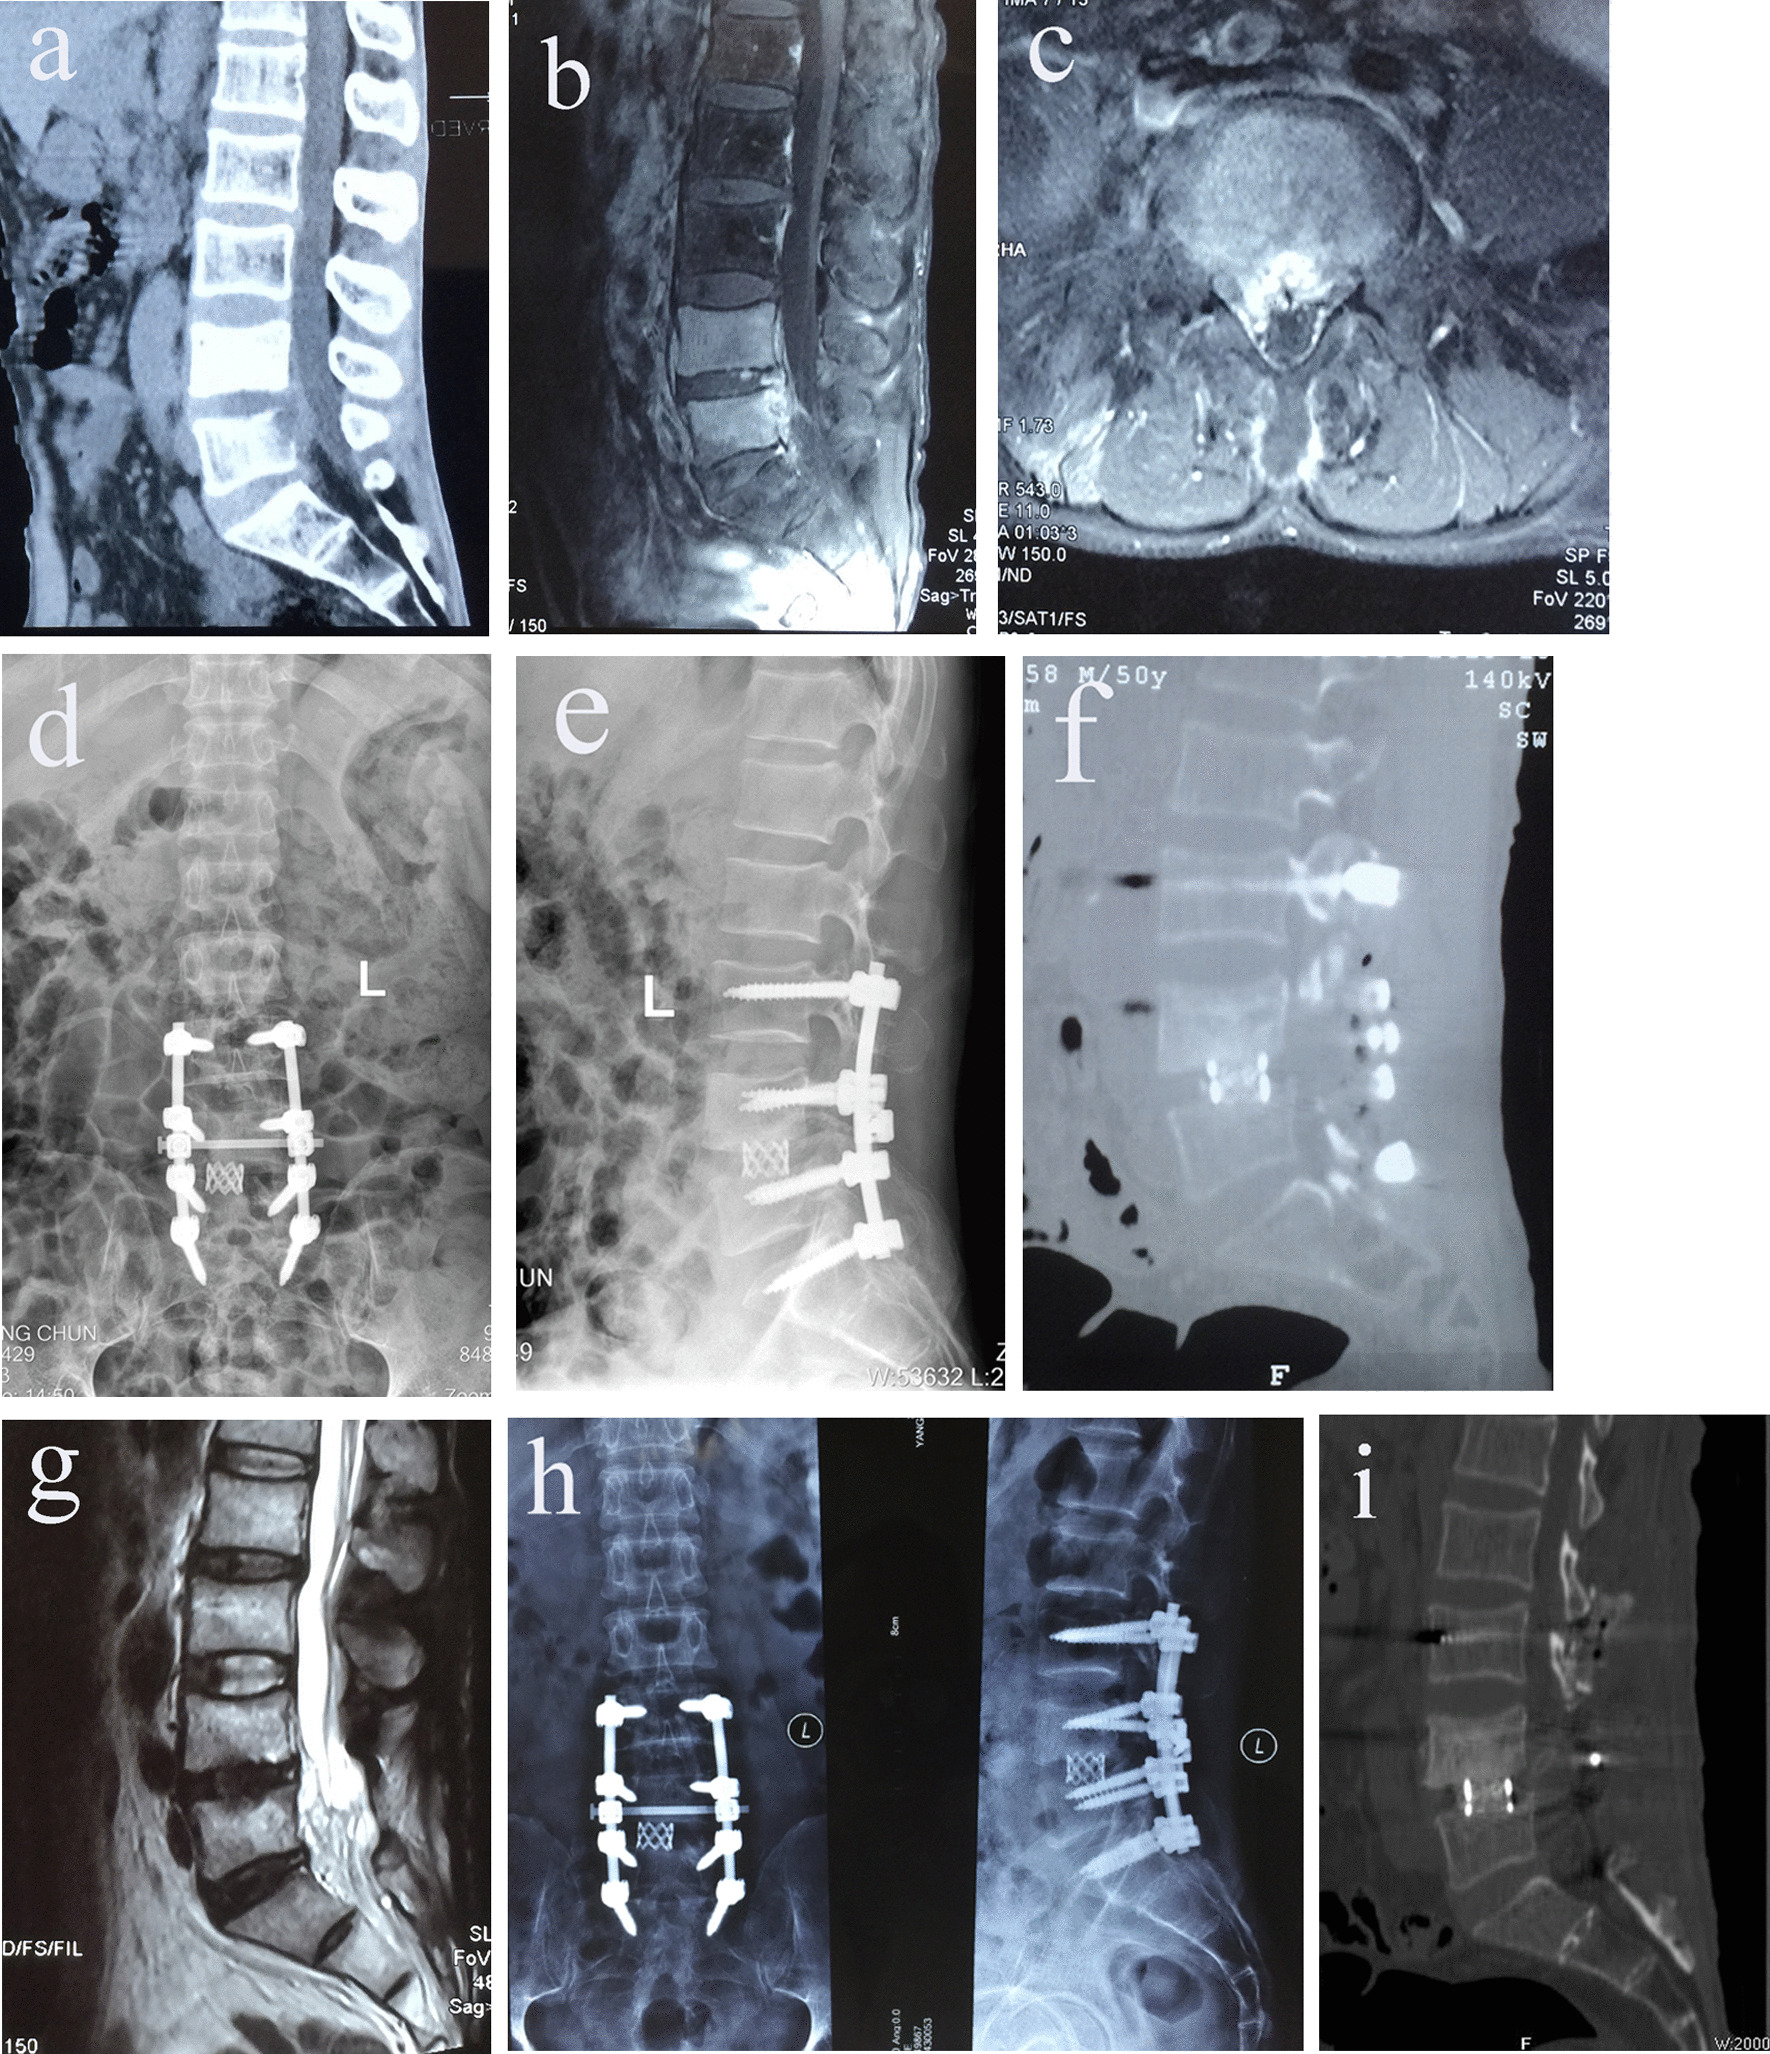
a–c Preoperative CT, and MRI showed that L4–L5 pyogenic vertebral osteomyelitis and lesion around vertebral body of L4–L5 developed paravertebral abscess with bony destruction.The abscess involved into the spinal canal with cord compromise resulted in neurologic deficit. d–g Postoperative radiographs and CT showed that interbody graft using titanium mesh cage, posterior short-segmental instrumentation and fusion. Postoperative MRI showed completely resolution of epidural abscess and decompression of neural component. h, i Final follow-up radiographs and CT showed good bone fusion
